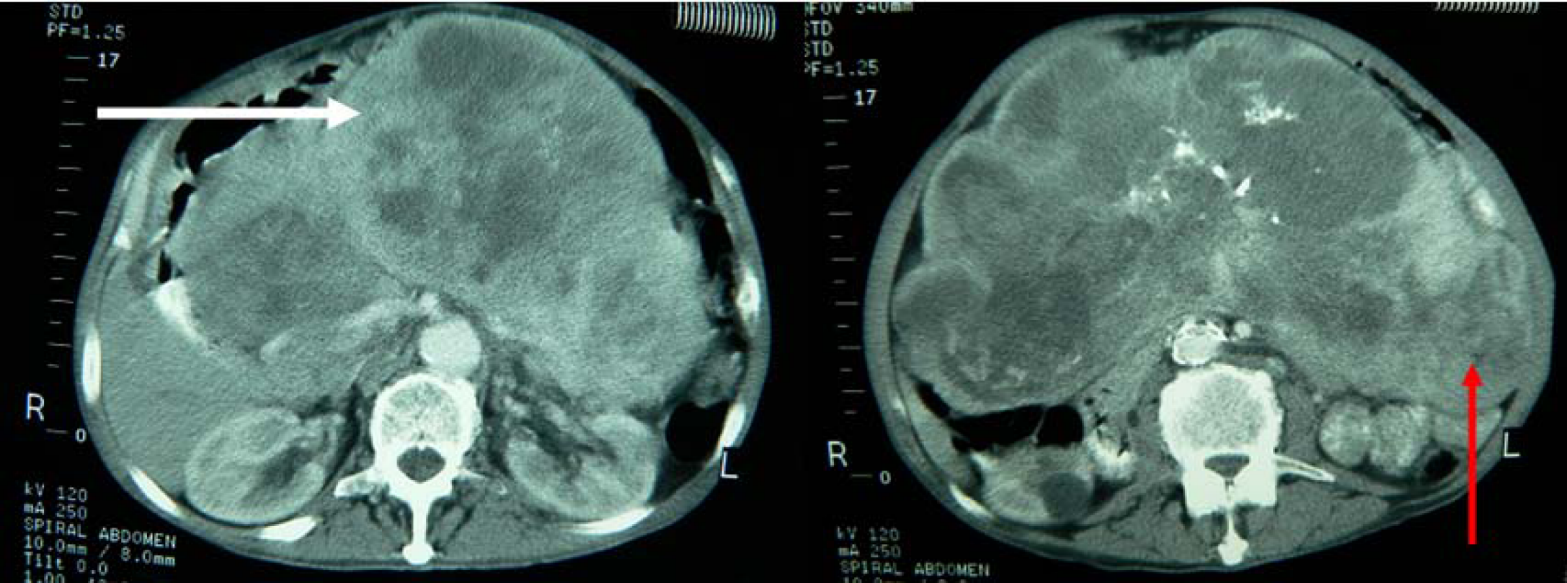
Preoperative spiral abdominal Computed Tomography images revealing mass formations (white arrow pointing to the large tumor and the red arrow pointing to the small tumor).
